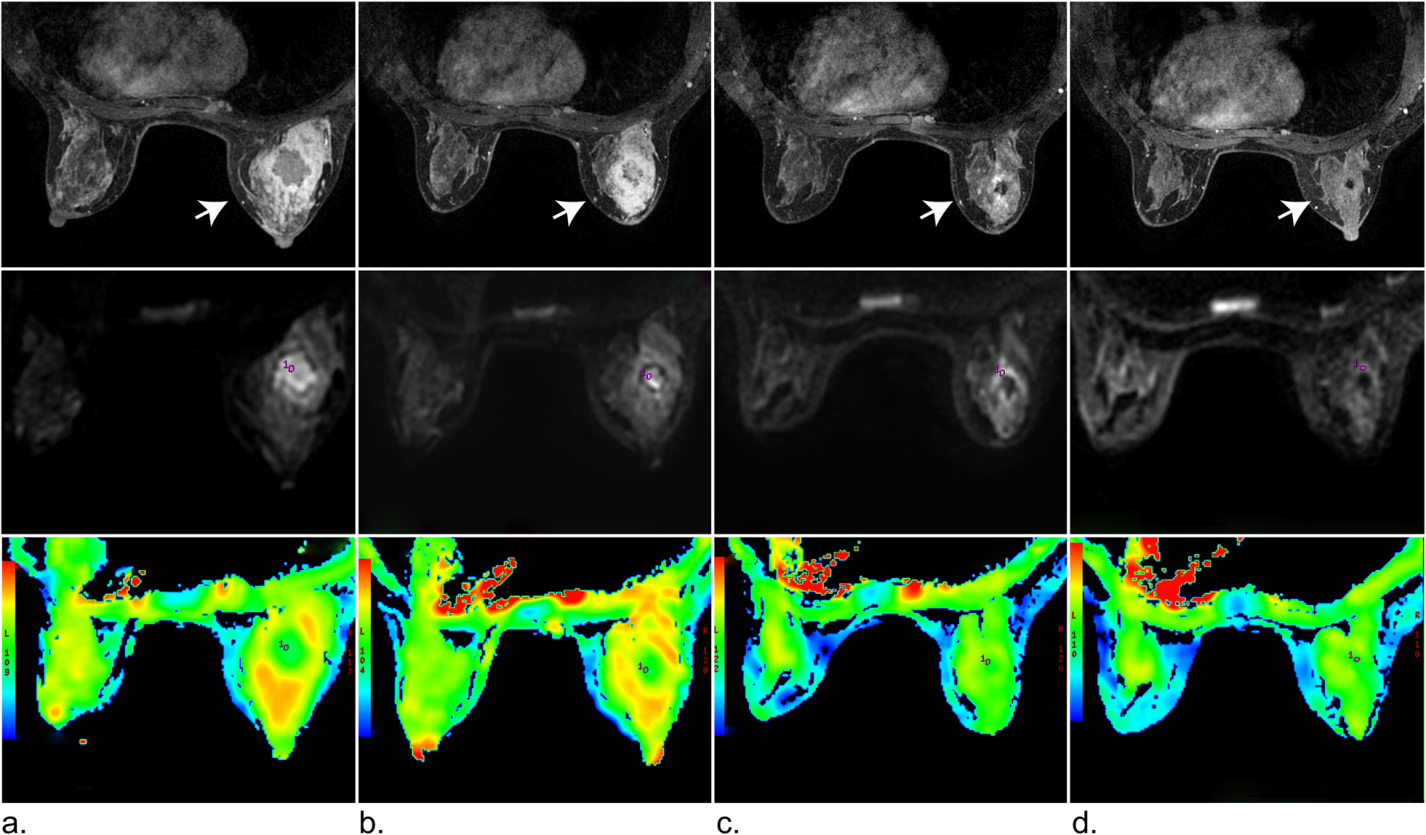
MRI images of a 49-year-old woman with invasive breast cancer who showed pCR after completing neoadjuvant chemotherapy (six cycles EC + T). a–d From top to bottom, the images show the vibrant enhancement sequence, IVIMnd ADC map, respectively. The ROI area of the DWI images is marked by a red circle. At baseline, first follow-up, second follow-up, and preoperatively, the tumor volume (white arrow) was 29.93, 22.44, 2.60, and 0.4 cm^2^, respectively, and standard ADC value was 1.0 × 10^−3^, 1.3 × 10^−3^, 1.5 × 10^−3^ and 1.3 × 10^−3^, respectively
